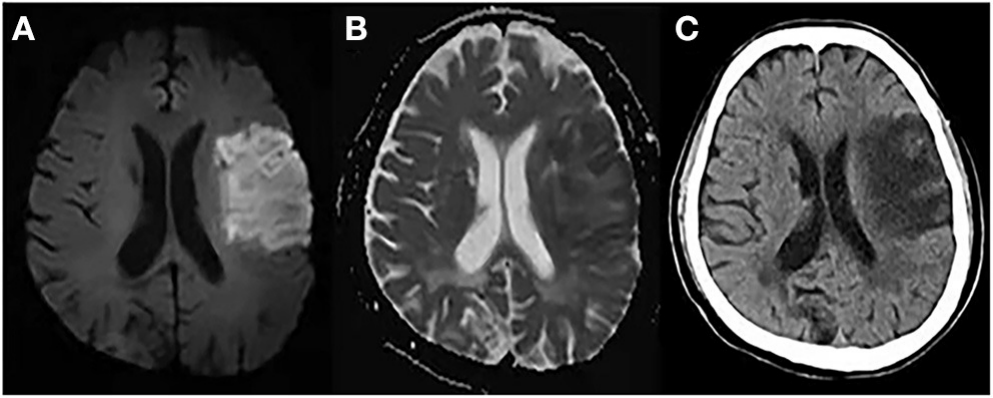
Cytotoxic edema, ionic edema, and vasogenic edema on MRI and CT. A 69-year-old male underwent MRI and CT on day 5 post-stroke. (A) DWI shows hyperintensity in the left frontal and parietal lobes. (B) ADC map shows hypointensity in the marginal area of the lesion, indicating cytotoxic edema, and hyperintensity in the lesion center, indicating ionic and vasogenic edema. (C) CT image shows hypodensity in the left frontal and parietal lobes, especially in the area of ionic and vasogenic edema (i.e., ADC hyperintensity area).
